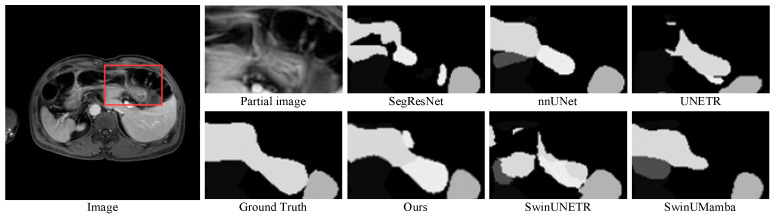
Enlarged view of the region indicated by the red box in the Abdomen MRI visualization.
